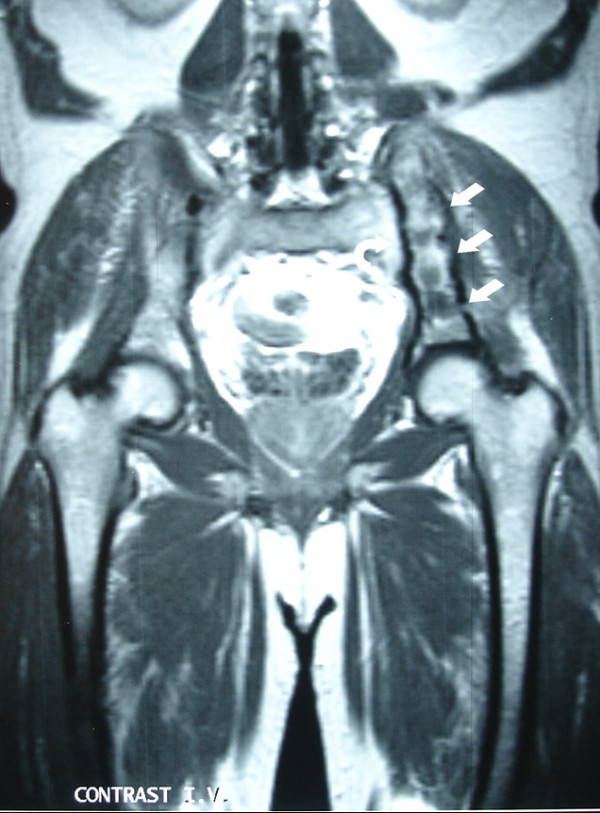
Postoperative post-contrast coronal T1-weighted MR image demonstrates diminution of the extension and the enhancement of the tumor at the left iliac bone (arrows) and at the left sacral wing (curved arrow).
